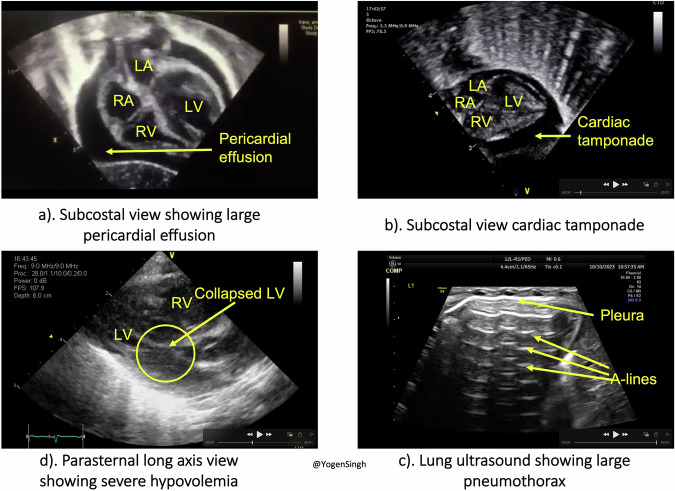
Ultrasound images acquired during neonatal resuscitation. Image a subcostal view showing large pericardial effusion, Image b subcostal view showing collapsed right atrium and right ventricle with pericardial effusion consistent with cardiac tamponade needing neonatal resuscitation – image acquired during chest compressions and then ultrasound guided pericardiocentesis performed, Image c showing a large pneumothorax with no lung sliding of pleura, excessive A-lines evenly spaced horizontal echogenic lines below the pleura) and no B-line (vertical echogenic lines extending below the pleura), and Image d showing a parasternal long axis view showing severe hypovolemia – “kissing sign” of left ventricle (collapsed left ventricle cavity).
